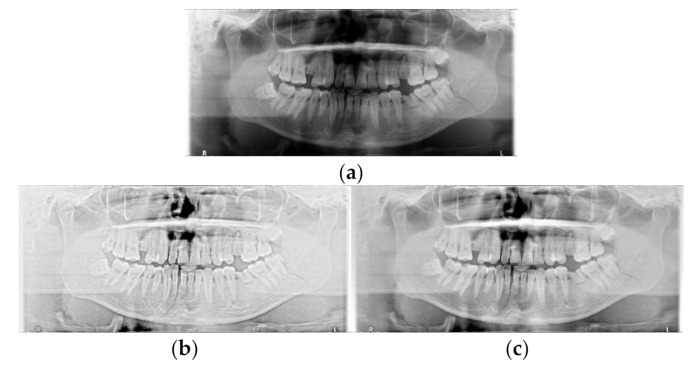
The comparison between original panoramic radiograph, single-scale luminance adaptation transform (SLAT), and multi-scale luminance adaptation transform (MLAT) panoramic radiograph: (a) Original, (b) SLAT, and (c) MLAT.
